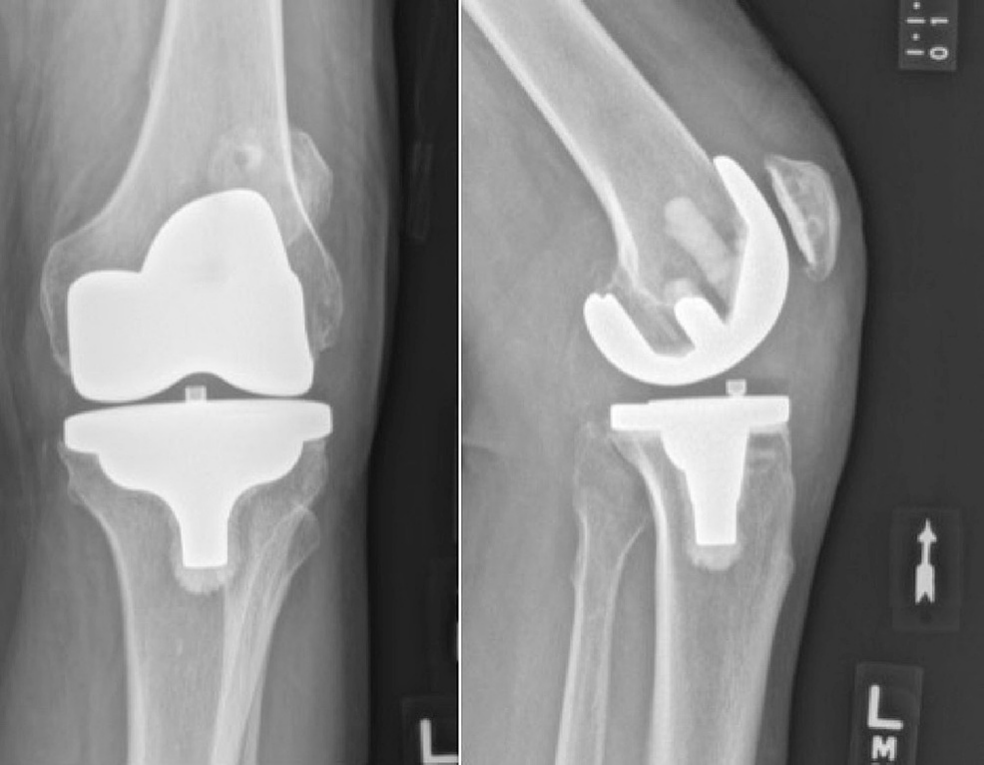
Anteroposterior (left) and lateral (right) radiographs obtained following the revision surgery with polyethylene swap and placement of the central tibial screw.
